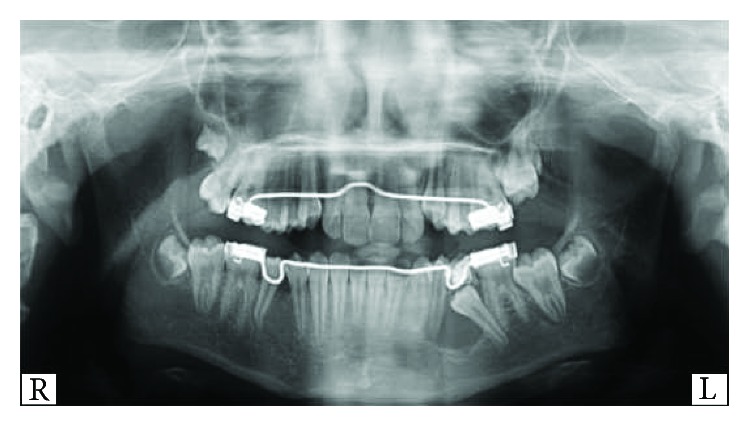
Orthopantomogram (OPG) showing a radiolucent lesion at the mandibular left side (second premolar region).
